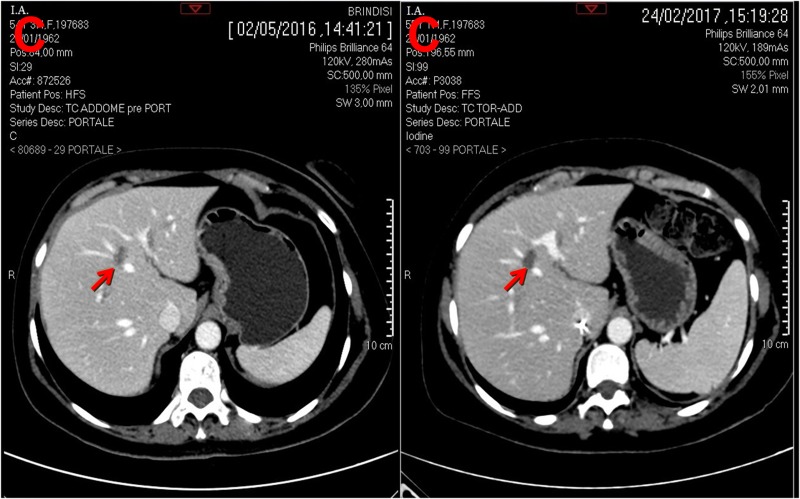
CT scan (portal phase) performed before and after hepatic artery infusion plus systemic chemotherapy a. Left arrow indicates LM at 4^th^ segment and right arrow indicates its complete disappearance at 4^th^ segment; b. Left arrow indicates LM at 5^th^ segment and right arrow indicates its complete disappearance at 5^th^ segment c. Left arrow indicates LM at 8^th^-4^th^ segment and right arrow indicates LM completely necrotic at 8^th^-4^th^ segment.
